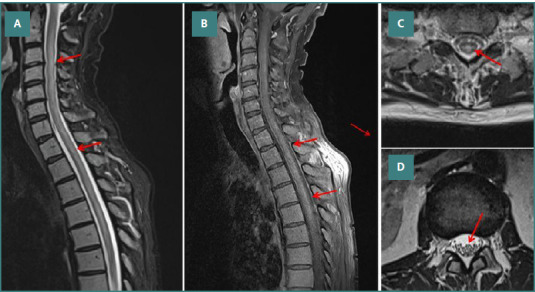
MRI of the spinal cord showing an (A, B) elongated, T2-hyperintense lesion C3 to T8 which mildly enhanced after application of contrast medium. C, D, on transverse sections the lesion was located centrally around the central canal
